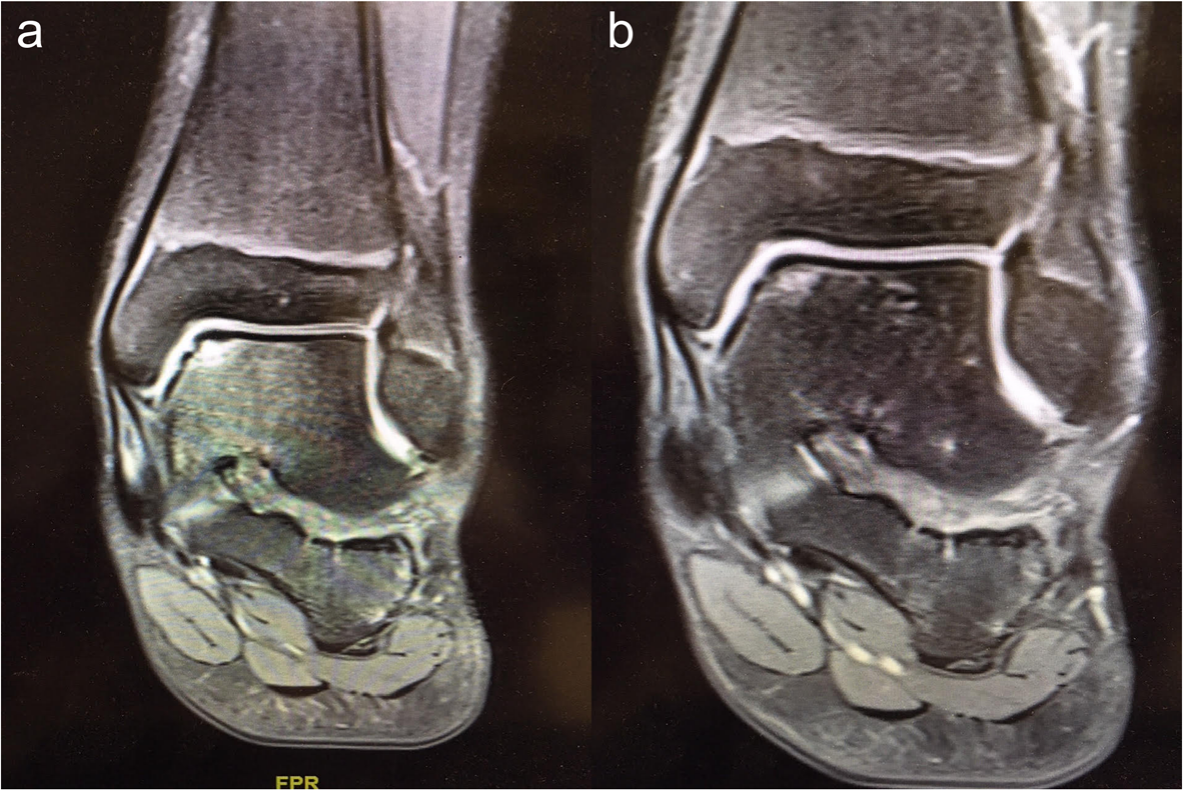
a/b MRI preoperatively (a) and 12 months postoperatively (b) in a 12 year old boy treated with retrograde drilling via electromagnetical guidance
